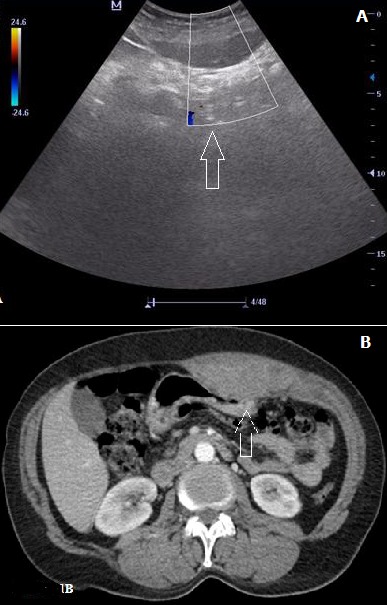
(A) bedside ultrasonographic view of the cystic mass in the anterior abdominal wall without blood flow; (B) enhanced CT scan showing a 3.5x4 cm fusiform dilatation in the left rectus muscle and diffuse increased density. Note the thickening of the abdominal wall
